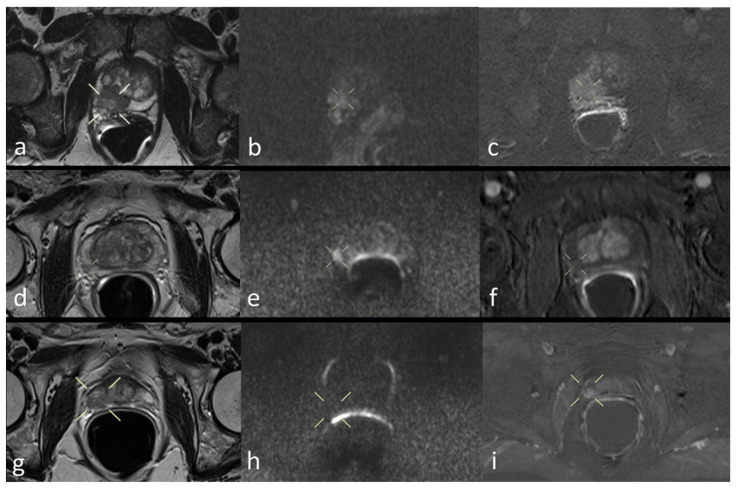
Prostate mpMRI of three patients: T2WI (a), DWI (b), and DCE image (c) in a true positive patient; T2WI (d), DWI (e), and DCE image (f) in a false positive patient; T2WI (g), DWI (h), and DCE image (i) in a false negative patient.
